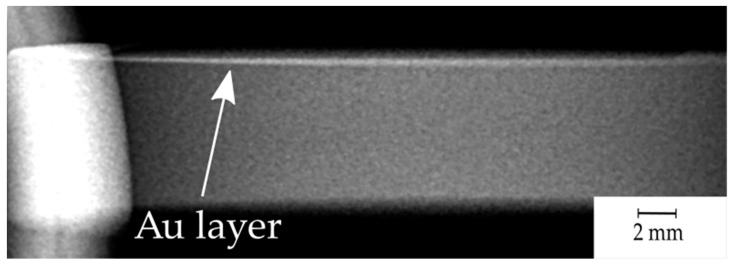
X-ray image (side view) of the sample with the revealed gold layer (top).
